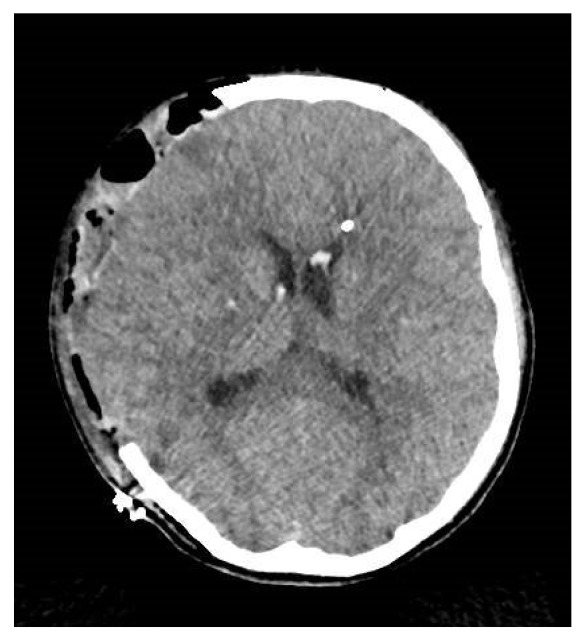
Cranial CT scan after decompressive hemicraniectomy, insertion of external ventricular derivation, and intracranial pressure catheter: cerebral swelling; hematoma on the right frontotemporal-parietal craniectomy.
